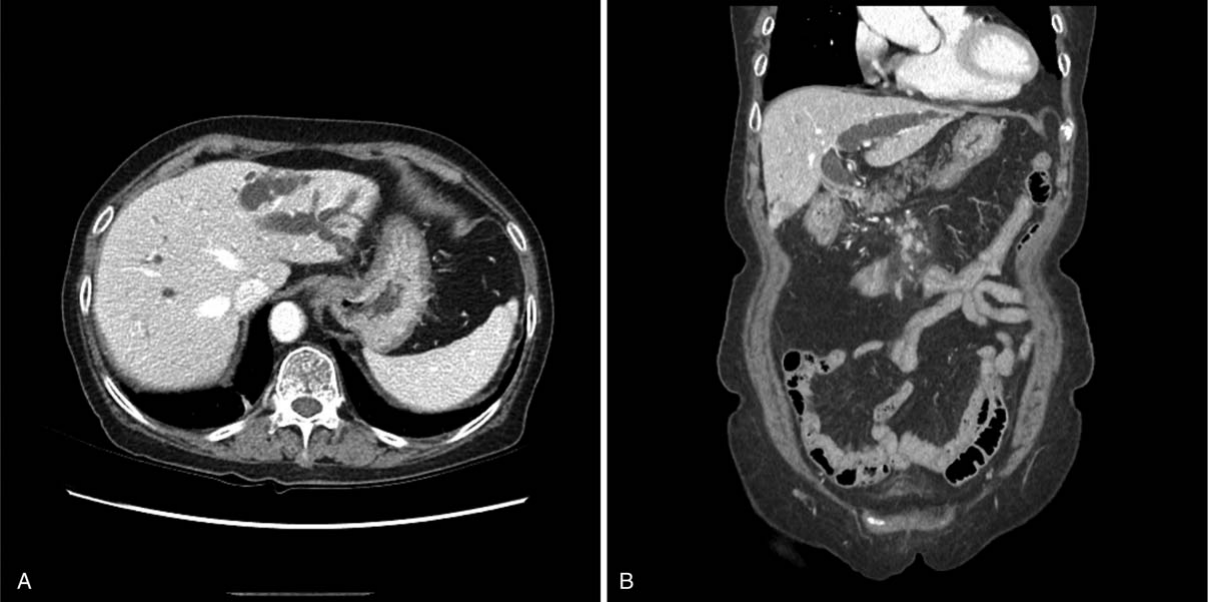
Abdominopelvic computed tomography showing dilatation of bile duct. (A) Axial view and (B) coronal view.
